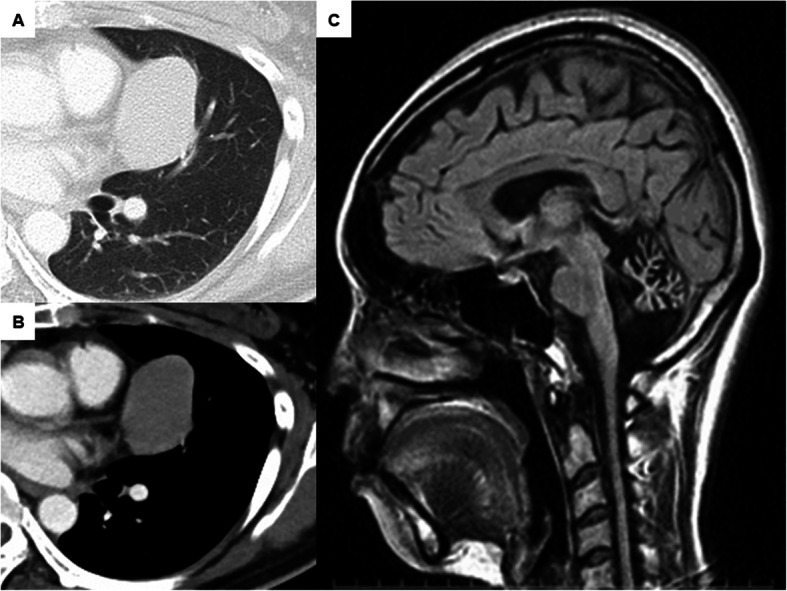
Imaging findings of the patents. Chest computed tomography shows (a) lung window image and (b) mediastinal window image of a well defined 48-mm mass in the left anterior mediastinum, in contact with pericardium and left lung. Sagittal weighted magnetic resonance image shows(c) severe cerebellar atrophy in a patient with spinocerebellar degeneration
